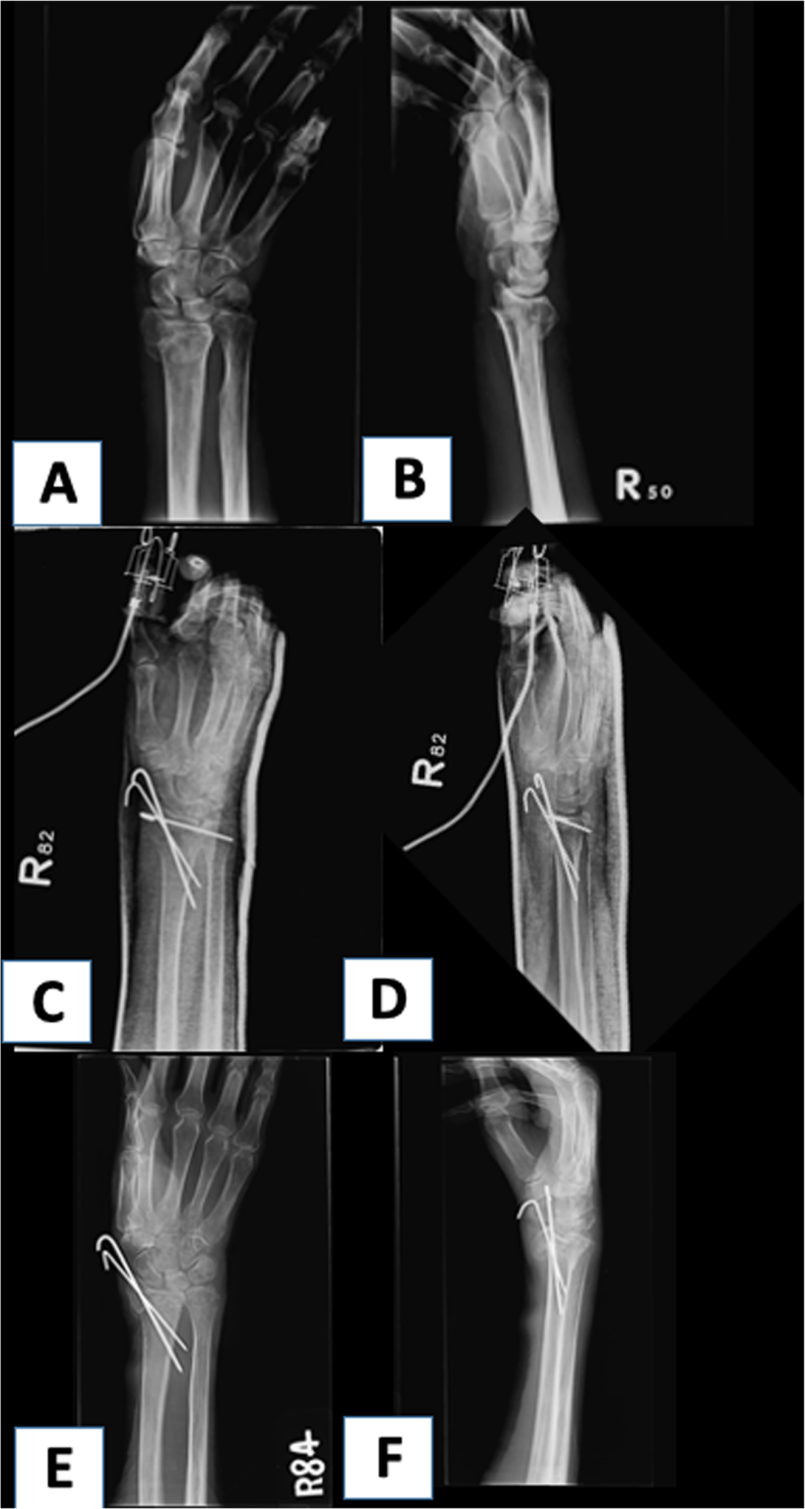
PD patient after k-wire fixation. This was a 67-year-old female that presented with right distal radial fracture and fixed with k-wires. a and b The preoperative radiographs. c and d Postoperative 2 weeks. e and f Postoperative 4 months showing loss of reduction
